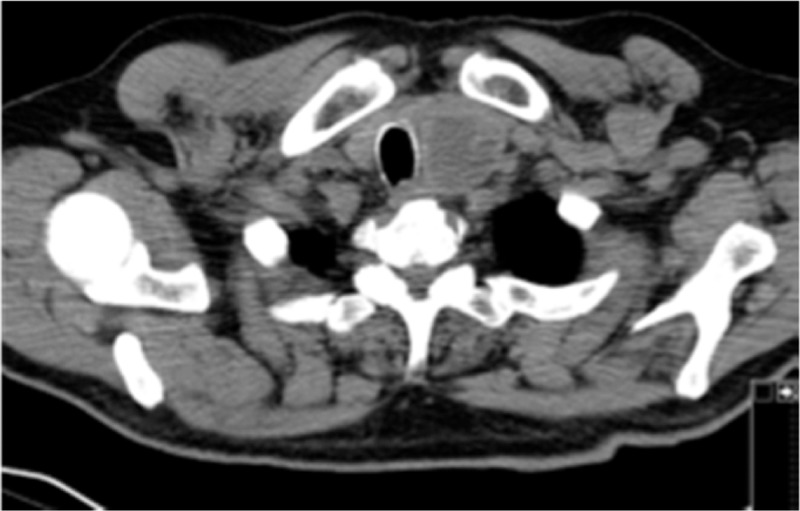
CT scan revealing tracheal deviation. Right tracheal deviation as seen in the figure could have led to irritation of the phrenic nerve promoting hiccups.
